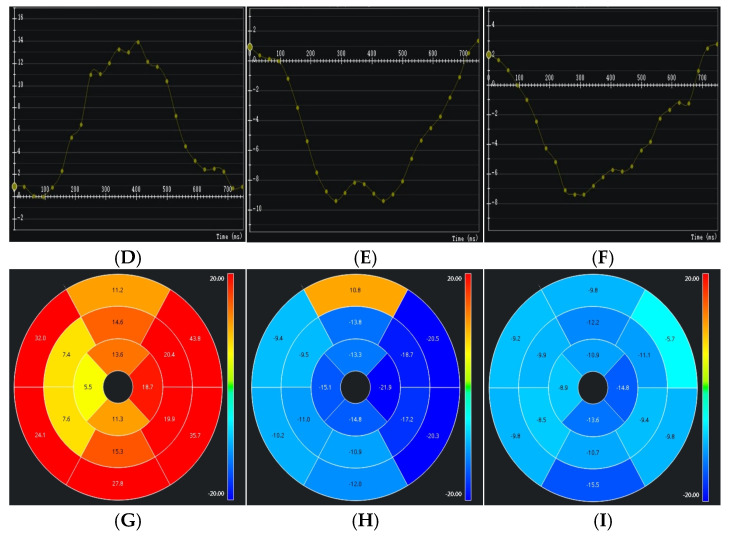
Example MRI studies of a 46-year-old man with NDLVC-rLVEF who had no adverse cardiovascular events at follow-up. (A–C): Left ventricular strain images in the short-axis, two-chamber, and four-chamber views, respectively. The contours of the left ventricular endocardium (red) and epicardium (green) are delineated. (D–F): Strain curves for 3D left ventricular global radial strain (GRS), global circumferential strain (GCS), and global longitudinal strain (GLS), respectively. (G–I): Sixteen-segment bull’s-eye maps displaying the distribution of GRS, GCS, and GLS, respectively.
